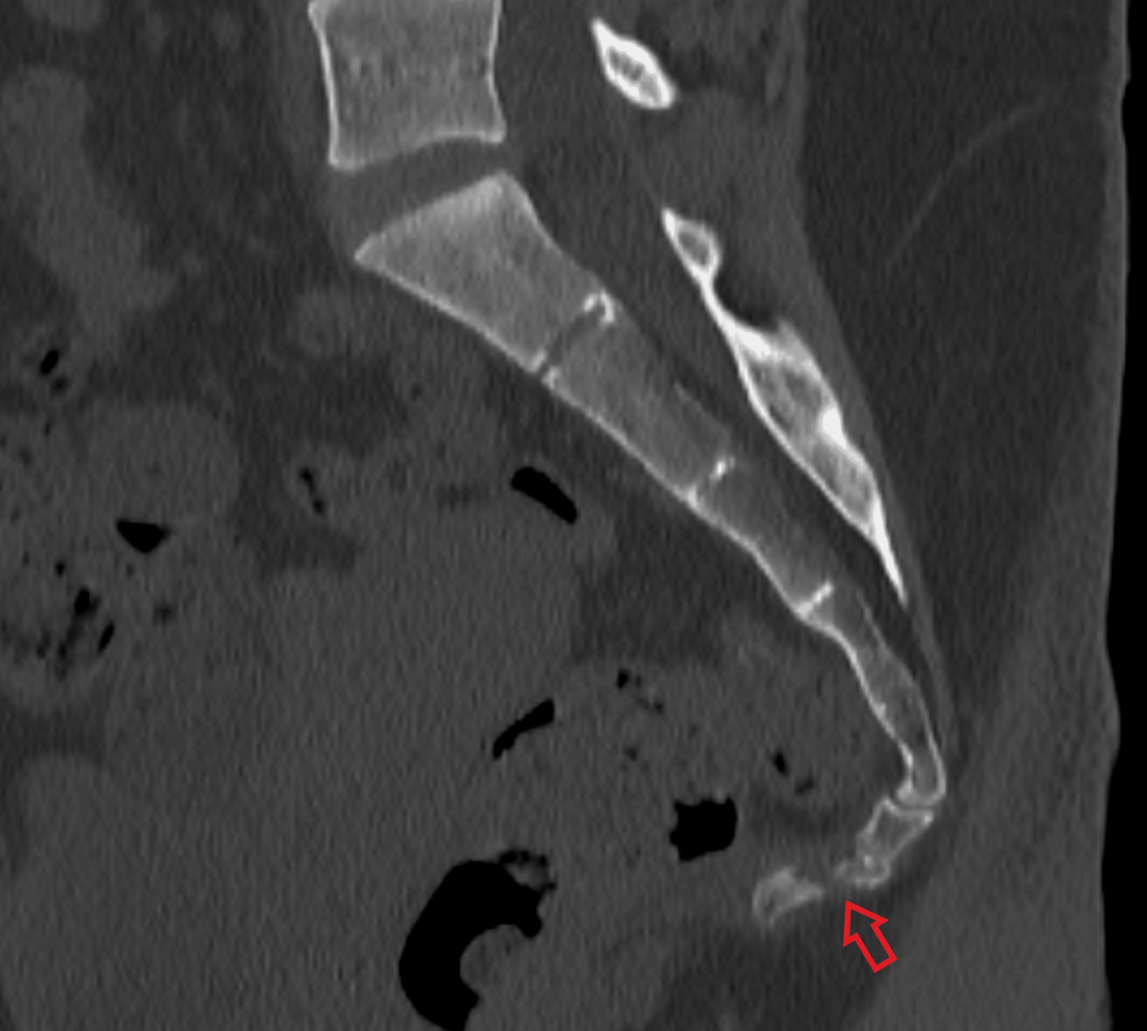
CT (sagittal view) of the coccyx Red arrow indicates transverse, complete, and anteriorly displaced fracture of the Cy2 segment of the coccyx, with traction measuring 3 mm.
